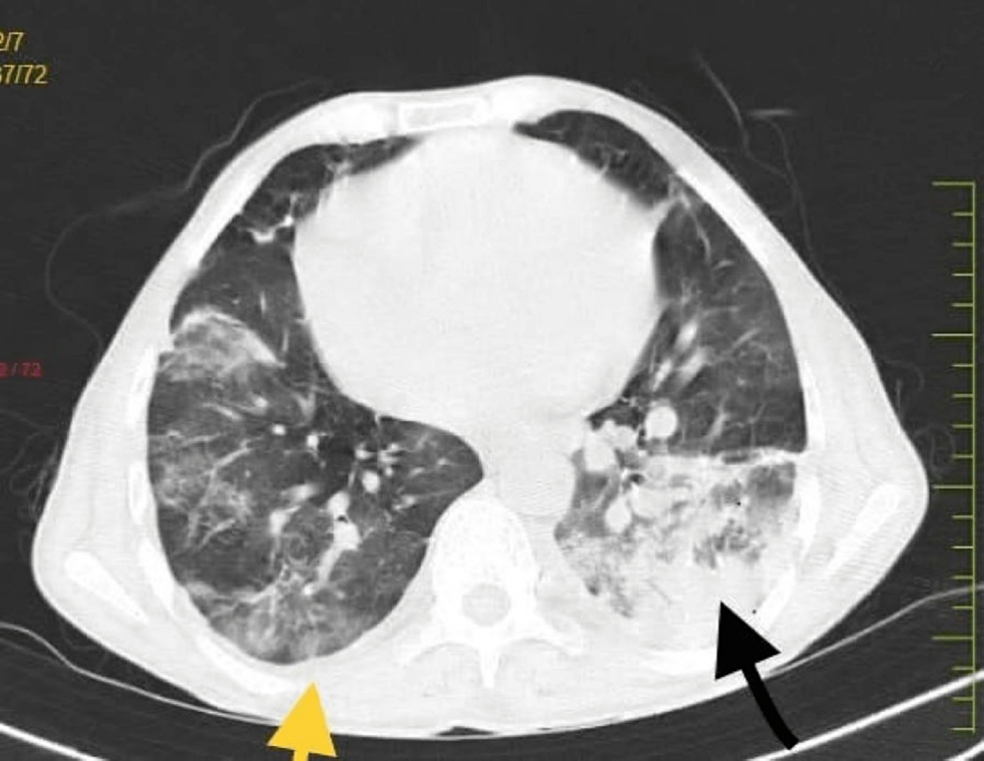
High-resolution CT scan of chest: the yellow arrow shows small right-sided effusion and the black arrow shows left lower lobe consolidation.
